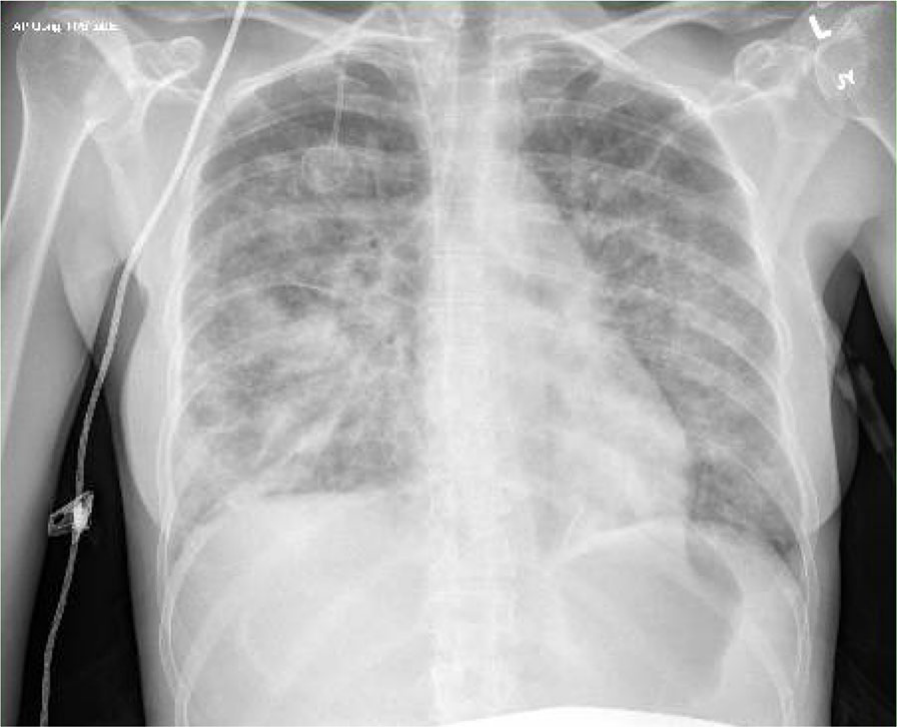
Frontal projection chest X-ray taken at onset of respiratory insufficiency, showing diffuse bilateral heterogeneous opacities
